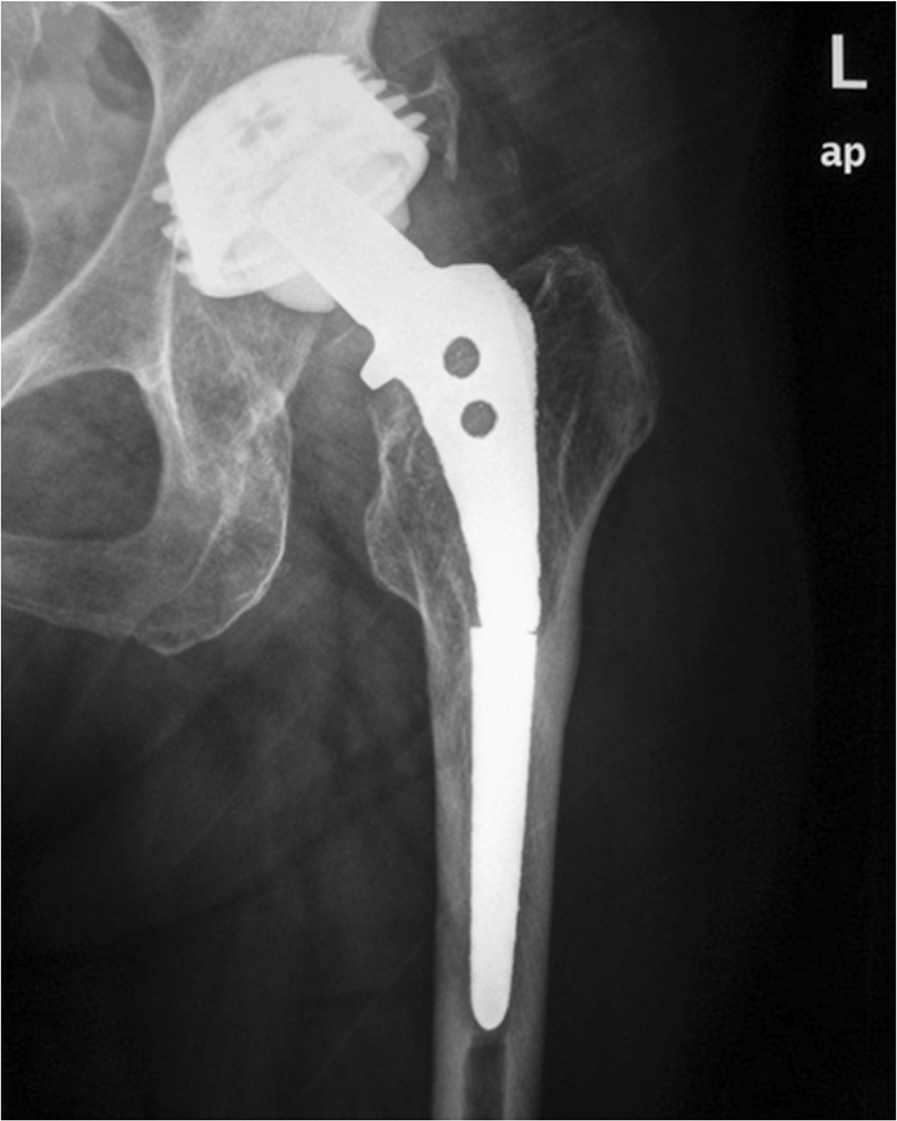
A case of a broken stem with a well-fixed proximal part. A 69-year-old female patient had an Autophor 900S stem implanted 15 years ago. This stem is entirely porous coated and has proximally two fenestrations for better anchoring. The arthroplasty became painful and an anteroposterior radiograph revealed a fatigue fracture of the middle of the stem. The distal part of the prosthesis seems firmly attached to the bone, whereas the stability of the proximal half is uncertain. During operation, this part could not be removed with standard techniques. Taking into account that distally the prosthesis is quadrilateral in cross section, it was decided to be revised with segmentation of the proximal femur.
